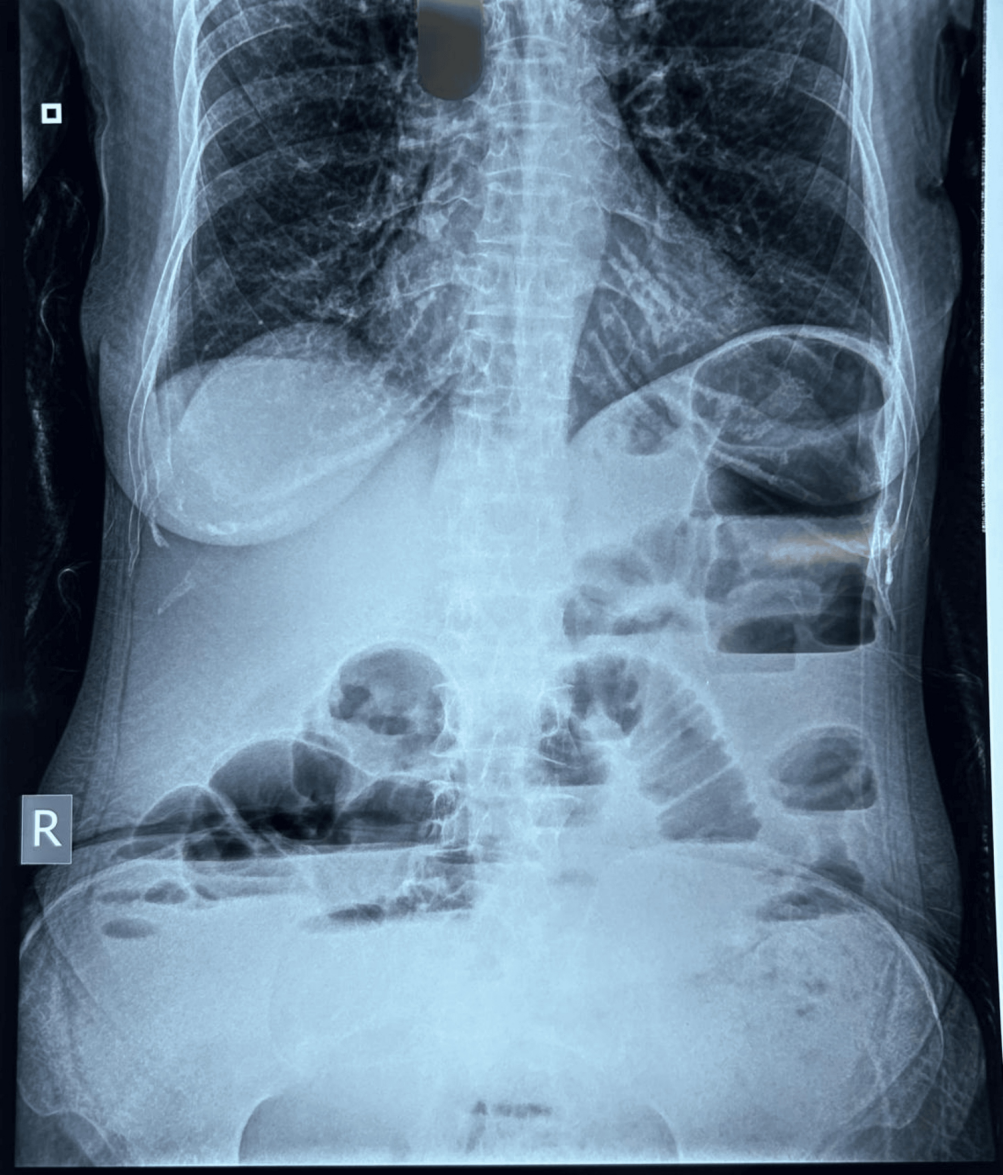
Abdominal X-ray of the patient showing multiple air-fluid levels suggestive of acute intestinal obstruction
